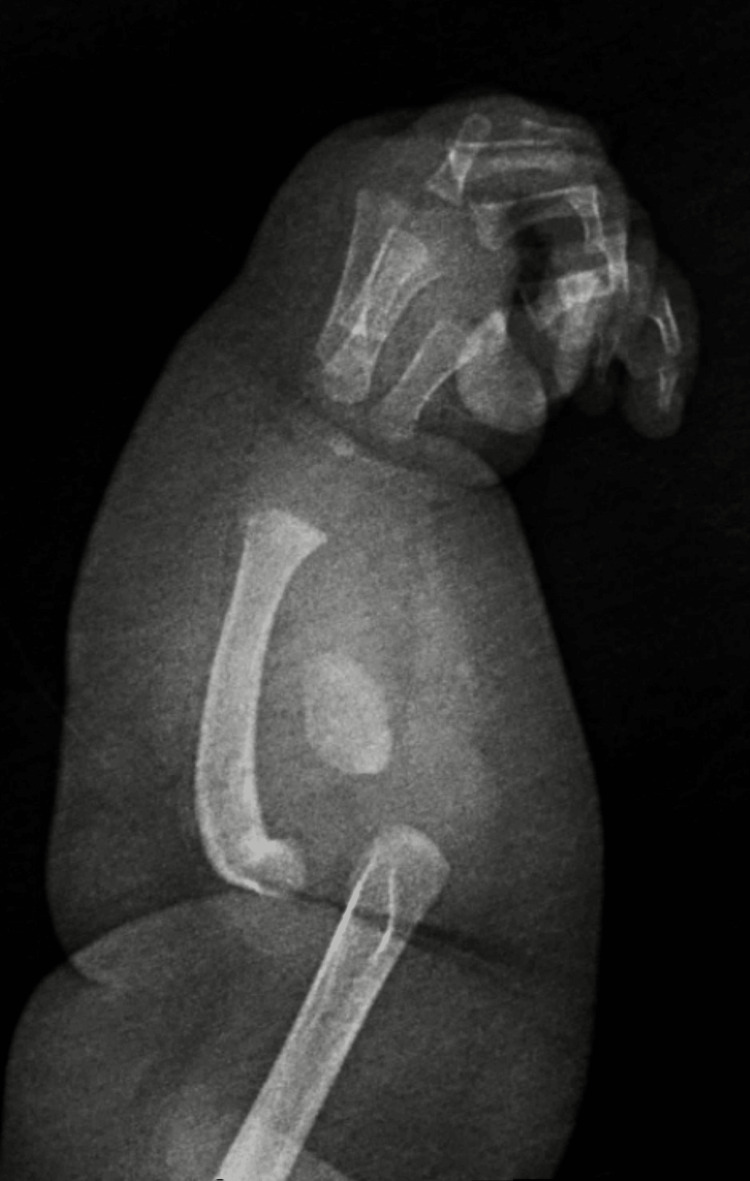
Left forearm and hand radiograph showing moderate bowing of the radius and frank dislocation with respect to the humerus.
